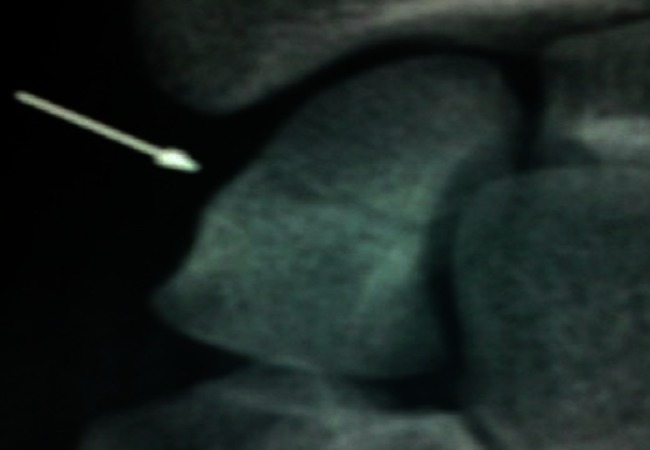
Radiographie du poignet de face centrée sur le scaphoïde objectivant une fracture corporéale non déplacée
